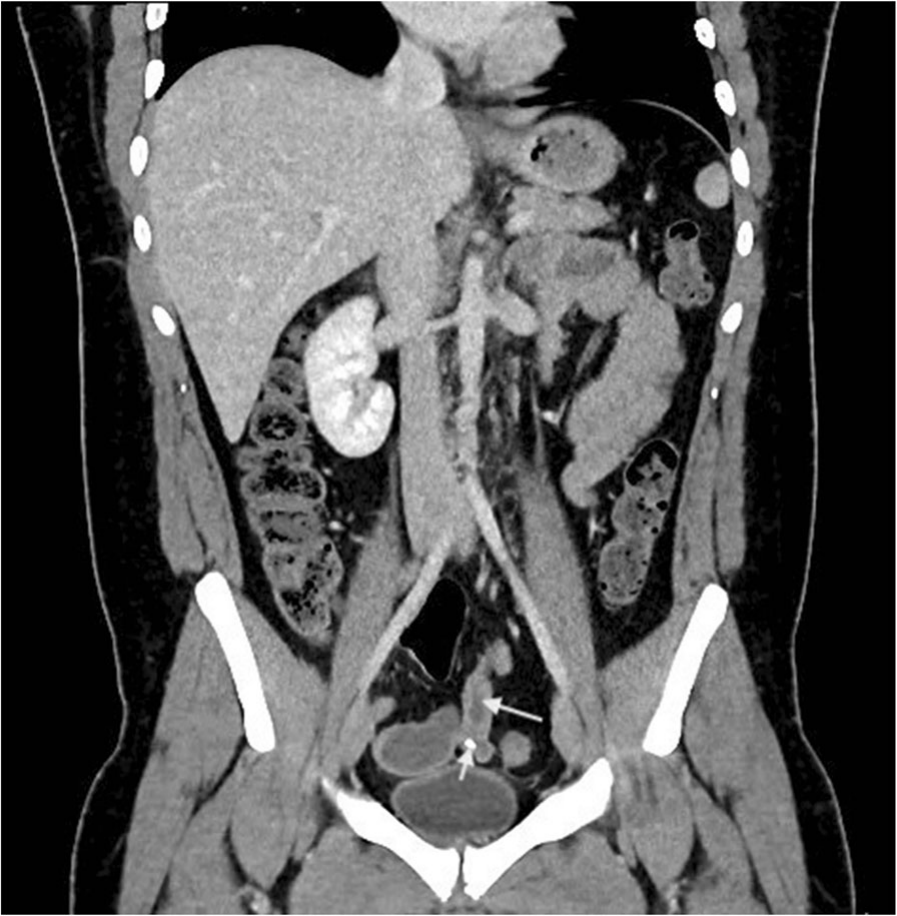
CT coronal view shows chicken bone (arrow head) inside MD (arrow)
